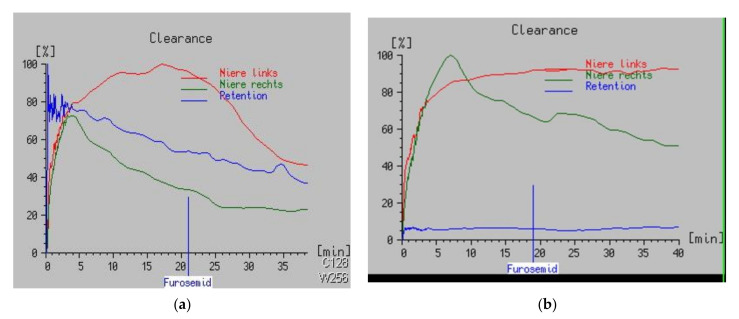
(a) In the kidney scintigraphy test, a retention kidney on the left side with borderline reaction on furosemide stimulation and excretion half-time of 17:39 min implicated a ureteropelvic junction obstruction (UPJO) (18 June 2020). Norm values: normal < 10 min, suspicious 10–20 min, abnormal > 20 min (courtesy of Dr. P. Zimmer, Klinikum Esslingen). (b) Repeated kidney scintigraphy test, constant retention kidney on the left side. Minor reduction of urinary clearence (left side 40%, right side 60%) (5 November 2020). Indication for surgery (courtesy of Dr. P. Zimmer, Klinikum Esslingen).
